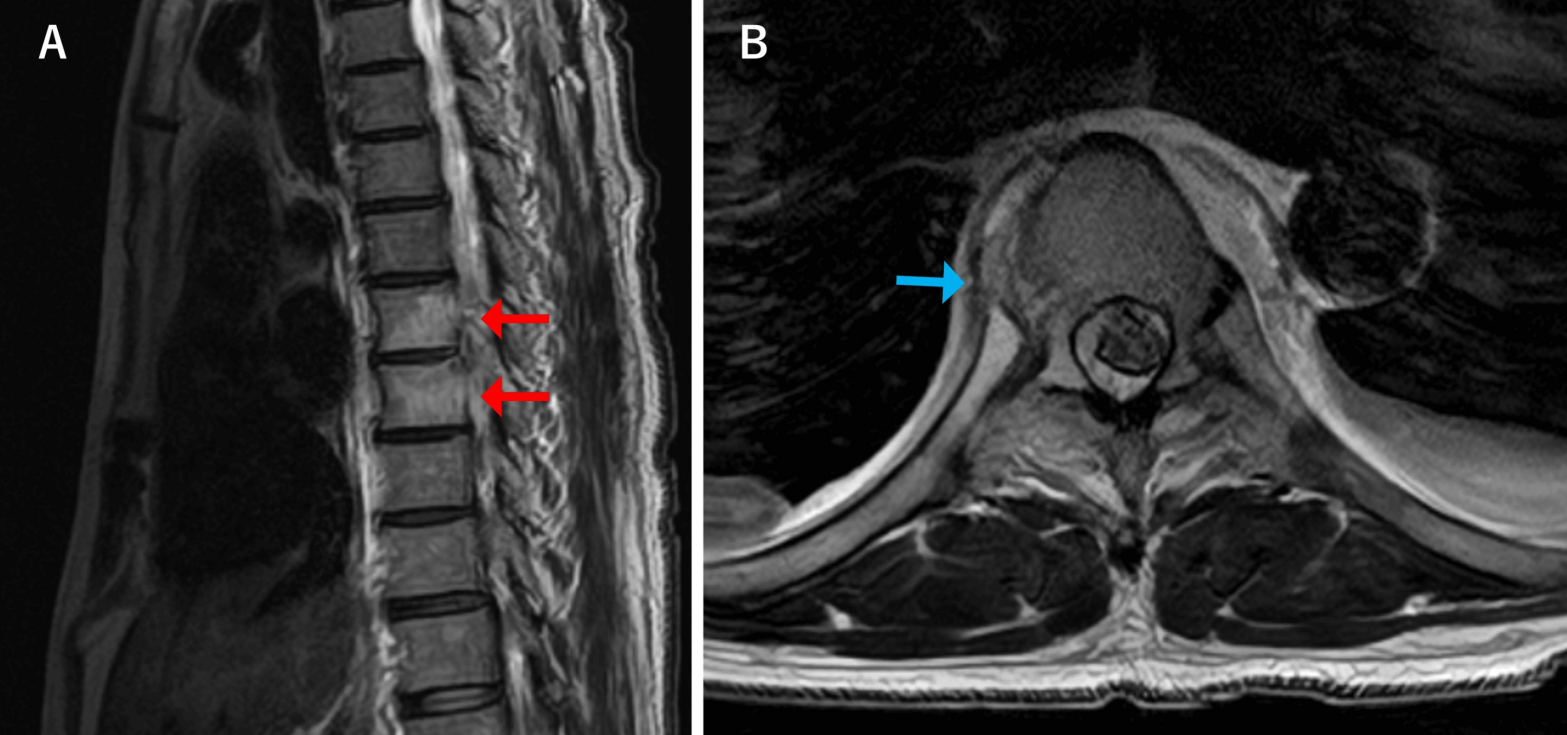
MRI images on the second day after admission MRI: magnetic resonance imaging (A) T2-weighted sagittal image shows a high signal intensity in the bone marrow of the T8-9 vertebral bodies (red arrows). (B) T2-weighted axial image shows a high signal area on the right side of the vertebral body at the T8-9 level (blue arrow)
